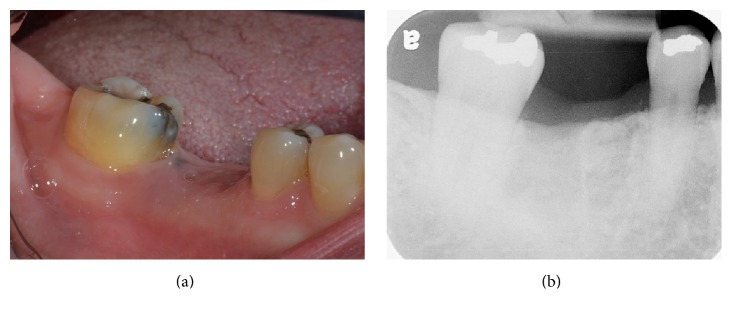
Preoperative views: (a) intraoral; (b) periapical radiograph.
